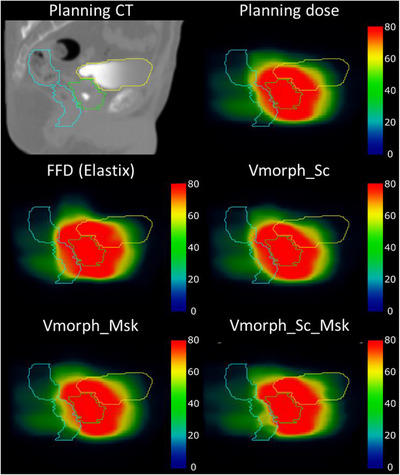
Qualitative evaluation of accumulated doses per DIR method for the overall median case regarding DSC. The first row contains the planning CT and dose. The second and third rows contain the accumulated dose per method with the planning contours. The green, yellow, and light blue colors represent the prostate, bladder, and rectum, respectively. The dose intensity parameters were 80 and 40 in Gy for the window and level, respectively.
